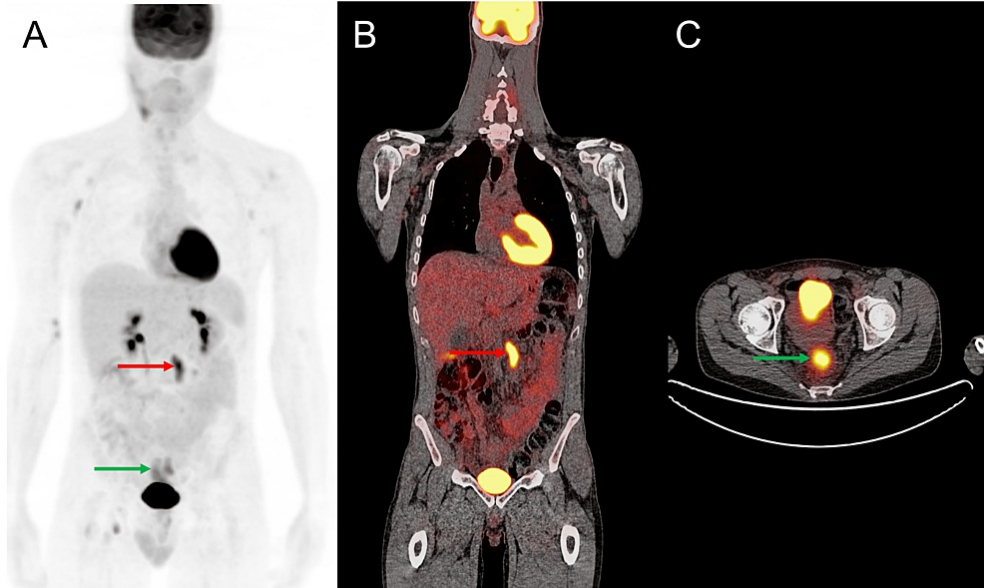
PET-CT images Maximum intensity projection (MIP) image of PET-CT (A) coronal-fused PET-CT (B) axial-fused PET-CT (C) demonstrating an F-fluorodeoxyglucose (FDG)-avid enlarged mesenteric lymph node with a maximum standardized uptake value (SUV) of 9.26 (red arrows). Additionally, there are FDG-avid rectal nodules with a maximum SUV of 16.33 (green arrows).
